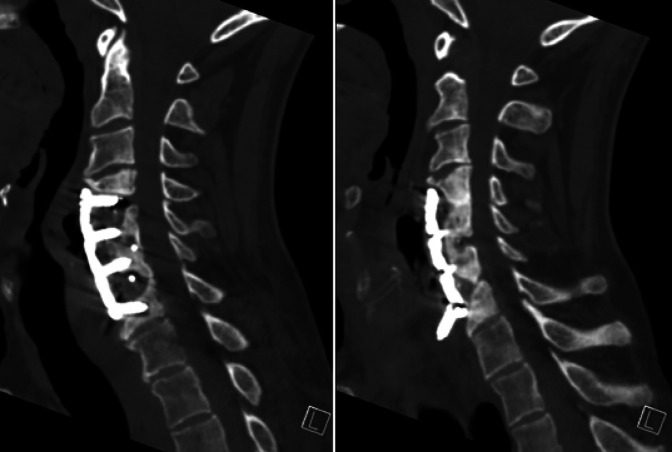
CT of the cervical spine 12 years after ACDF demonstrating pseudoarthrosis at C4-5 and C6-7, erosive changes to the vertebral bodies of C4-5 and C6-7 with loss of bone directly posterior to the plate, loosening around the left C7 screw (left image), anterior displacement of the right C7 screw (right image), and fluid/air anterior to the surgical plate. ACDF = anterior cervical diskectomy and fusion
